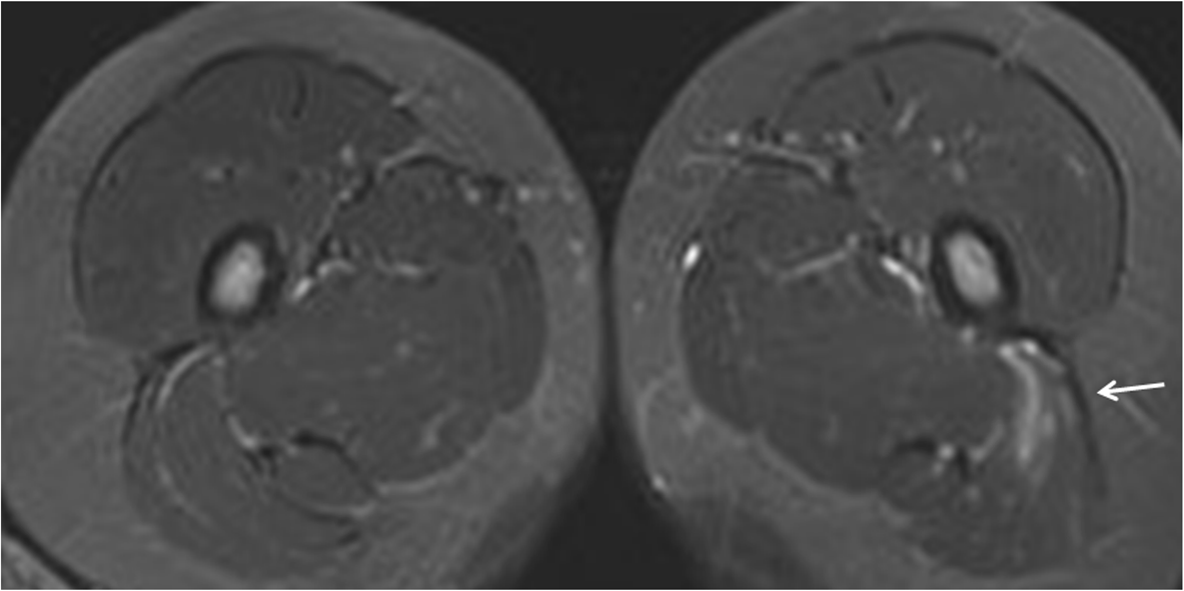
Enthesitis of the left gluteus maximus insertion in an 8-year-old girl with enthesitis-related arthritis on an axial STIR MR image (arrow)
